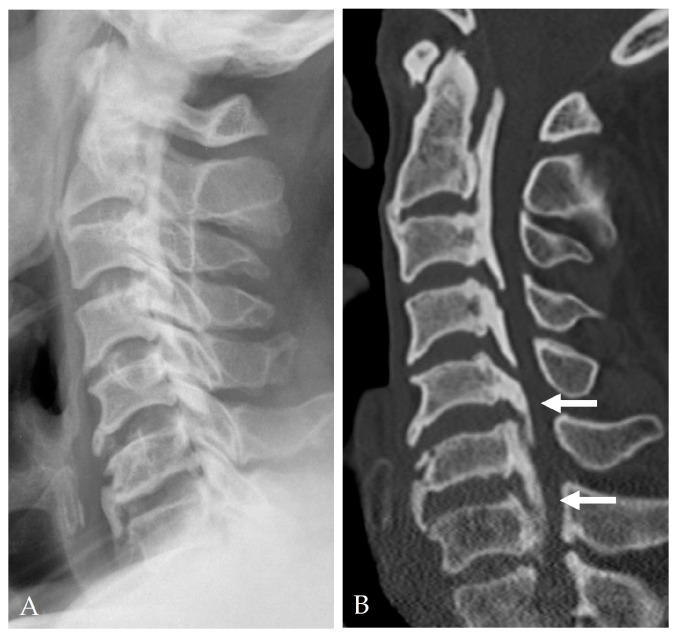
Lateral X-ray and 2-dimensional sagittal reconstructed CT showed ‘negative K-line’ OPLL (white arrows) (A,B).
